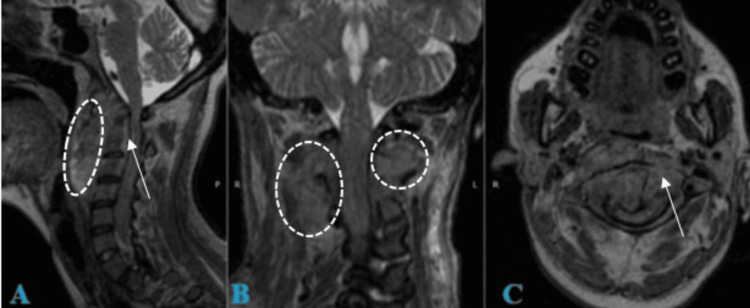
T2 MRI of the cranio-cervical junction and cervical spine A. Sagittal view: Shows a retro-pharyngeal infiltrative process with intradural, extradural, extramedullary invasion. There is an obstruction of cerebrospinal fluid (CSF) flow from the prebulbar cistern and cisterna magna into the cervical dural sac. B. Coronal view: Demonstrates infiltration of the lateral masses from C2 to C4 and adjacent soft tissues. C. Axial view at the C1 level: The odontoid process is centered within the anteroposterior diameter of C1, with evidence of ventral displacement of C1 and pannus infiltration. There is an absence of CSF hyperintensity surrounding the spinal cord.
